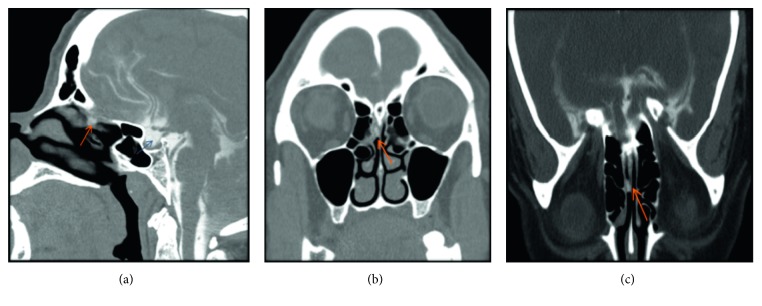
Sagittal (a), axial (b), and coronal (c) images in prone position with scanning during sneezing maneuver. CT cisternogram demonstrated leakage of intrathecally injected contrast (red arrow) noted in the right nasal cavity, along the right aspect of the perpendicular plate of nasal septum, just below the right cribriform plate. Empty sella filled with intrathecally injected contrast (blue arrow).
